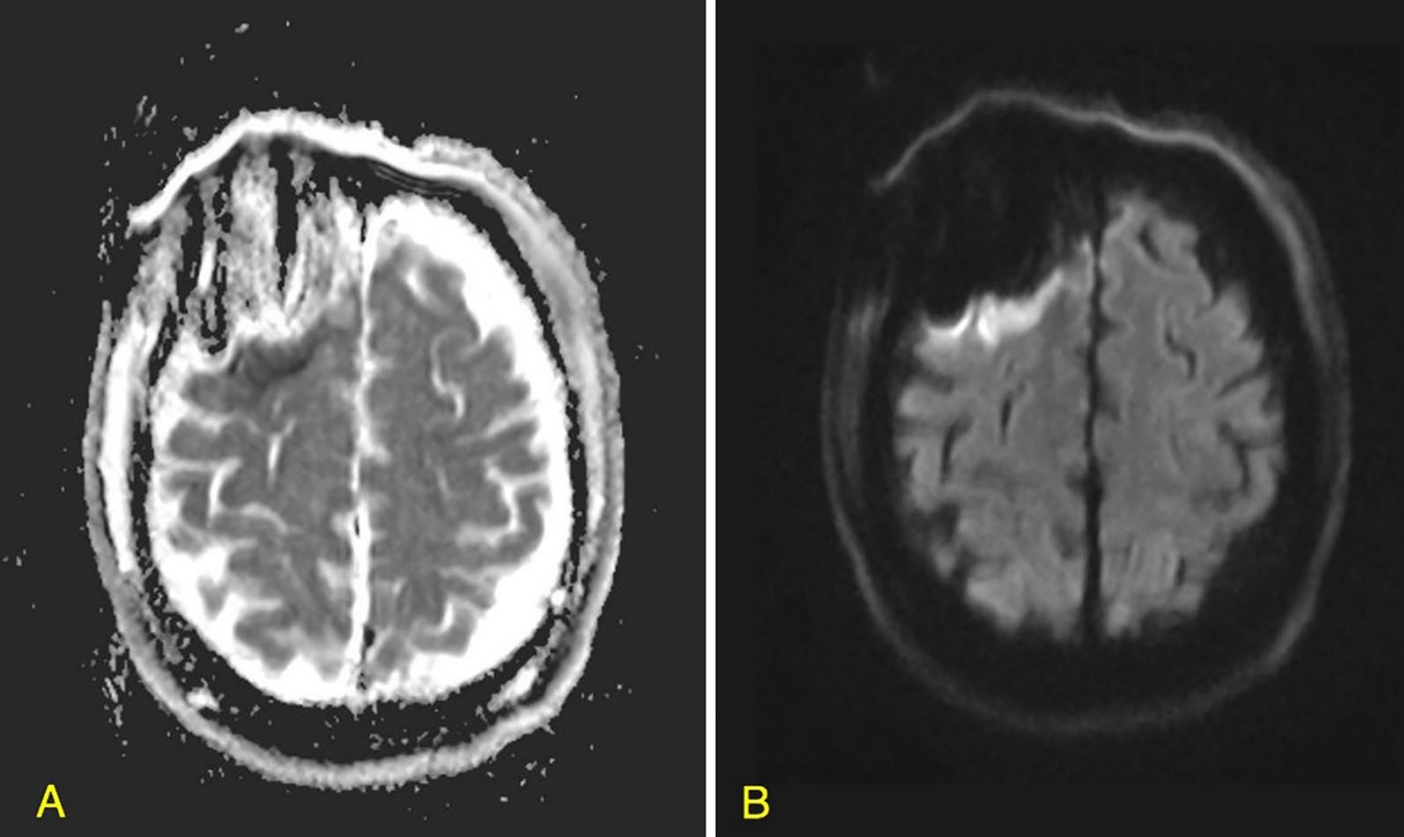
Post-up MRI imaging of a 52 year old patient after resection of GBM, with peri-resection rim restriction and no post-op neurological deficits. (A) Apparent-diffusion coefficient (ADC) sequence; (B) diffusion-weighted imaging (DWI) sequence.
